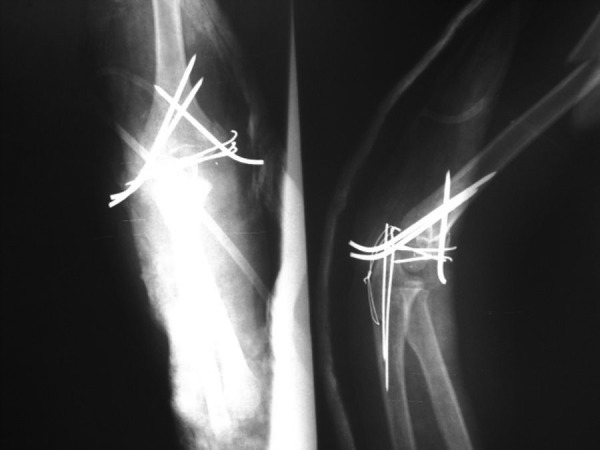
AP and Lat X-Rays After Open Reduction and Internal Fixation of the Fractures
